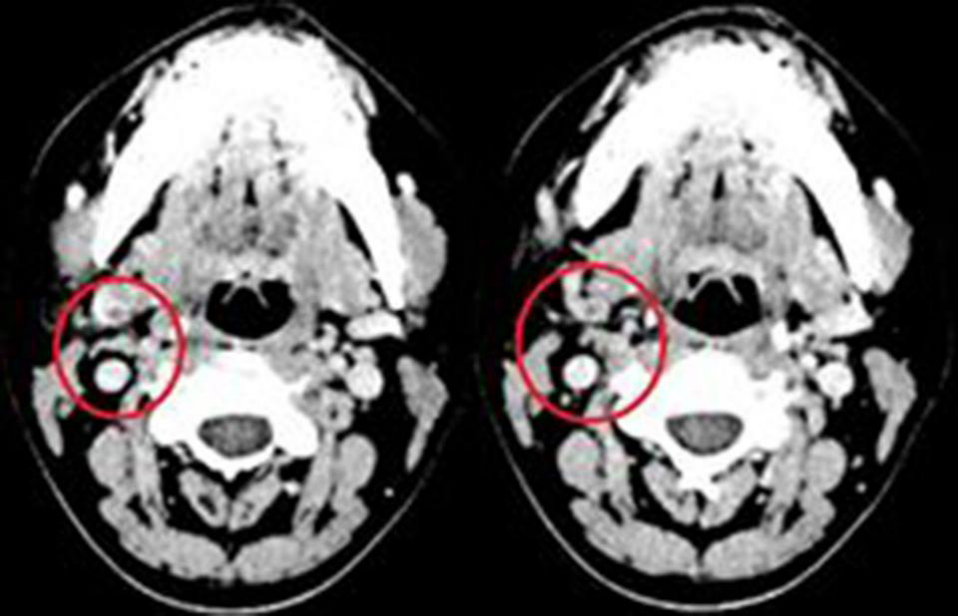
Sequential computed tomography slices showing areas of thrombophlebitis of right internal jugular vein branches (inside red circles).
